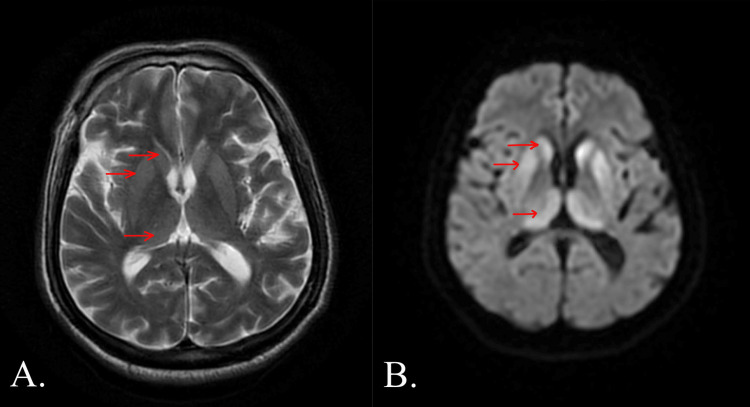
Non-contrast enhanced MRI BRAIN: A. Axial T2-weighted image and B. Axial DWI image, with arrows showing near-symmetric hyperintensities showing diffusion restriction noted in bilateral caudate lobes, anterior aspect of putamen and dorsomedial thalami MRI: Magnetic resonance imaging DWI: Diffusion-weighted imaging
